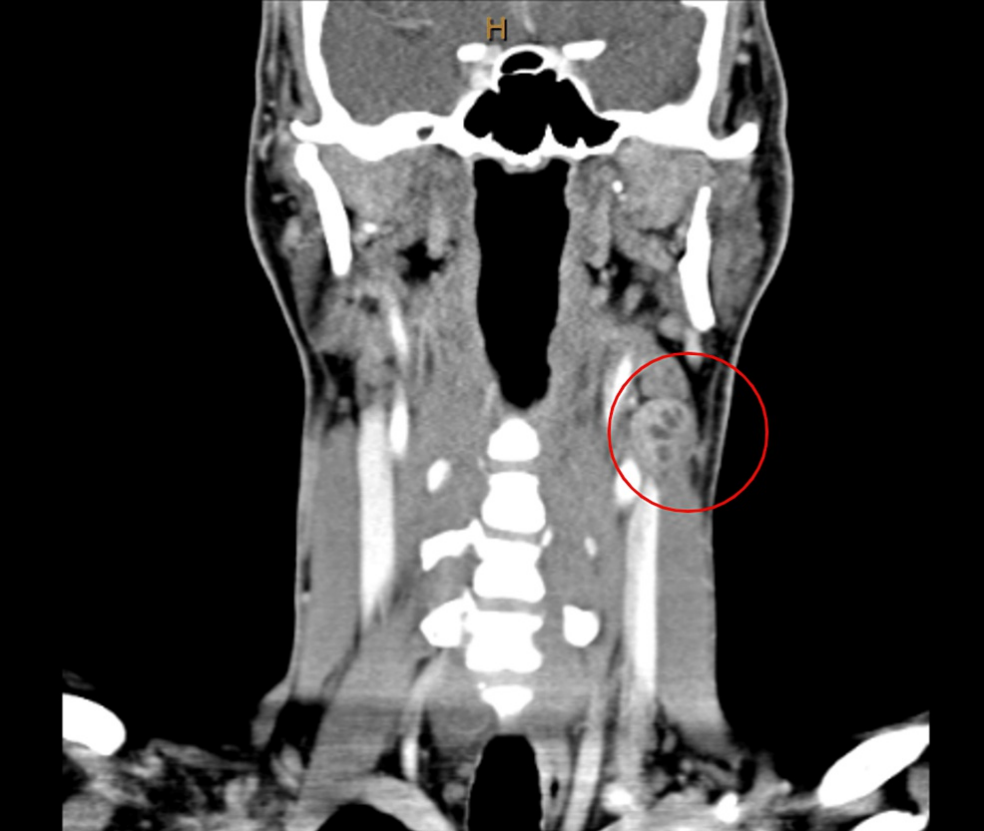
Initial neck coronal view computed tomography scan with and without contrast The red circle demonstrates the mass of interest
